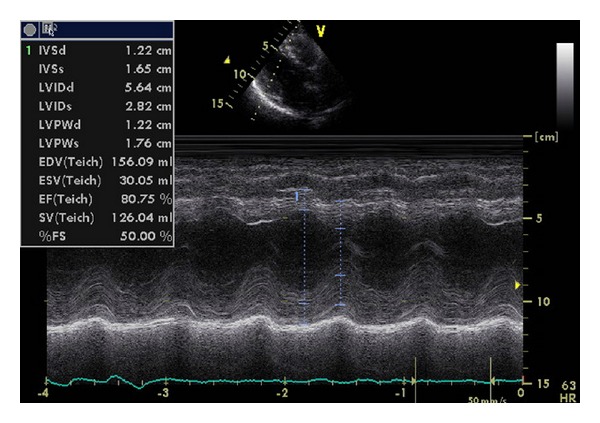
Case 1; echocardiography after bortezomib.
